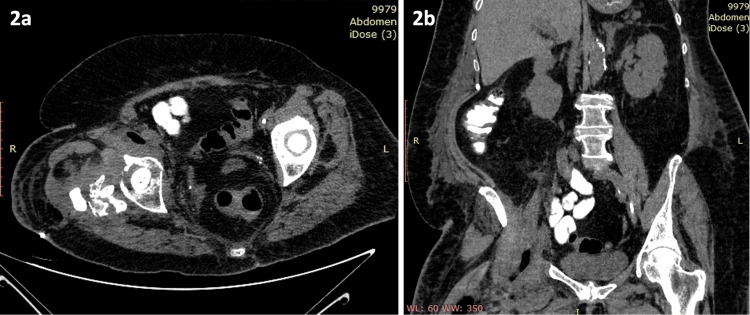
Postoperative CT scan. Transverse (2a) and coronal (2b) views of postoperative CT scan showing various empty spaces in retroperitoneum and hip region created by abscesses drainage.
